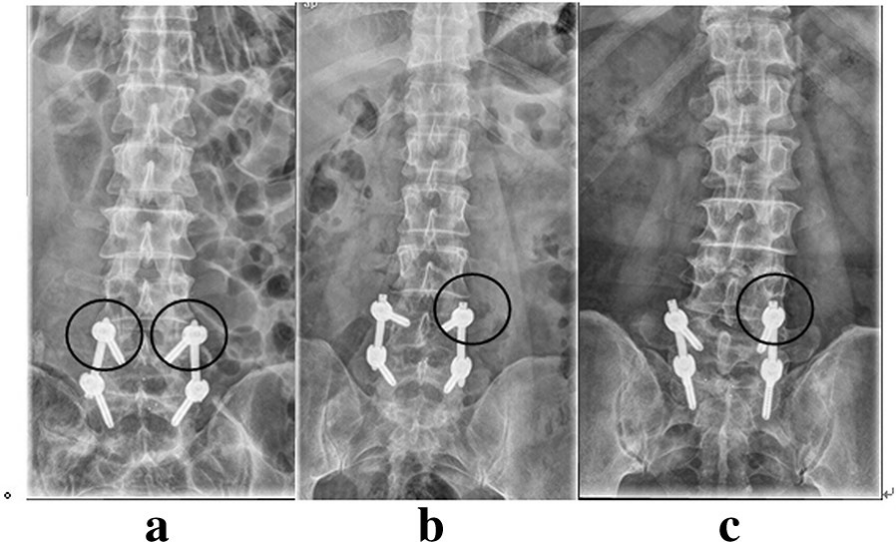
Postoperative X-ray images of patients. a X-ray image of 0 point facet joint violation; b X-ray image of one point facet joint violation; c X-ray image of two point facet joint violation
